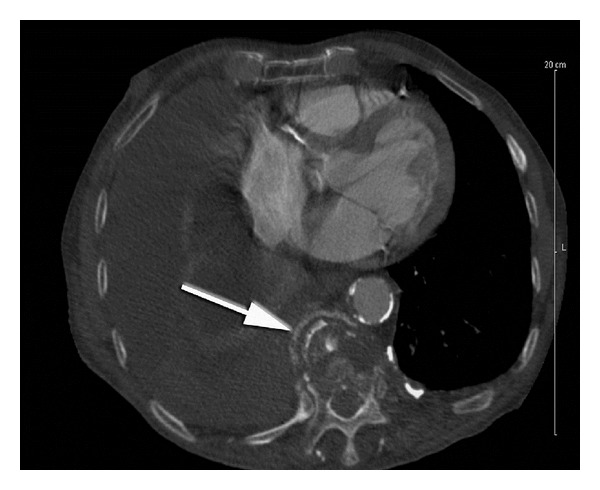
CT of case report 1 showing location of thoracic fracture.
